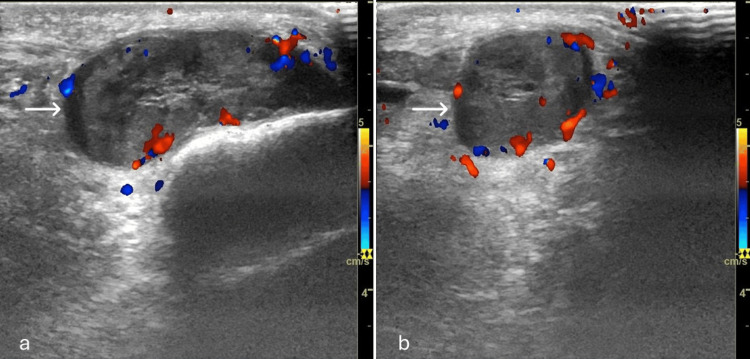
Ultrasound images of the left knee joint: (a) longitudinal and (b) transverse Showing a well-defined oval-shaped solid soft tissue lesion (arrows) in the antero-lateral infrapatellar region measuring (4 cm × 1.6 cm). The lesion is of mixed echogenicity and showed internal vascularity. There are no calcifications/cystic changes.
